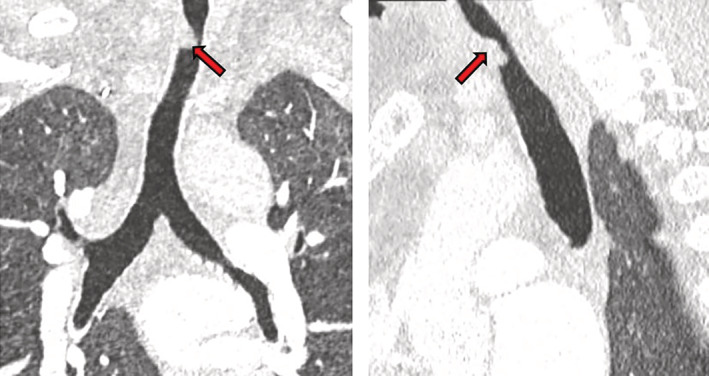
Axial and coronal CT scan views. Excess granulation tissue formation around the tracheal stoma site (arrow), responsible of a tracheal stenosis estimated to 40%.
